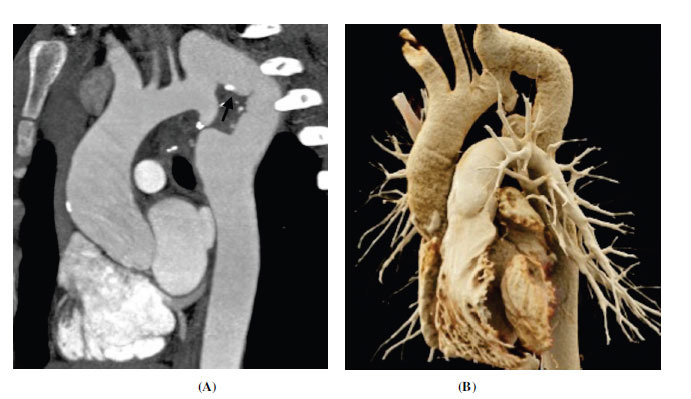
Aortic pseudo coarctation (A) Sagittal contrast-enhanced computed tomographic angiography image and (B) Cinematic rendered image tortuosity of the aortic arch at the level of isthmus. No collateral vessels are seen. Echocardiography showed no gradient across the tortuous segment suggesting pseudo coarctation.
